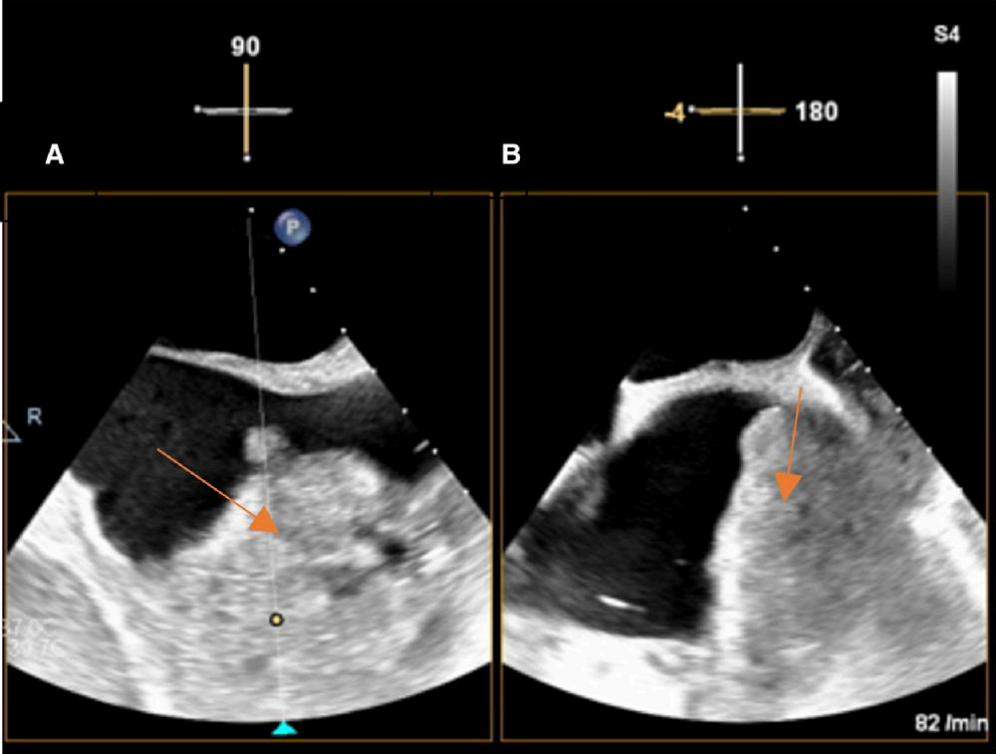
Transoesophageal echocardiogram at 90° in the bicaval view (*A*) and corresponding orthogonal plane (*B*) in the multiplane echocardiogram showing the large mass filling out large parts of the right atrium.
